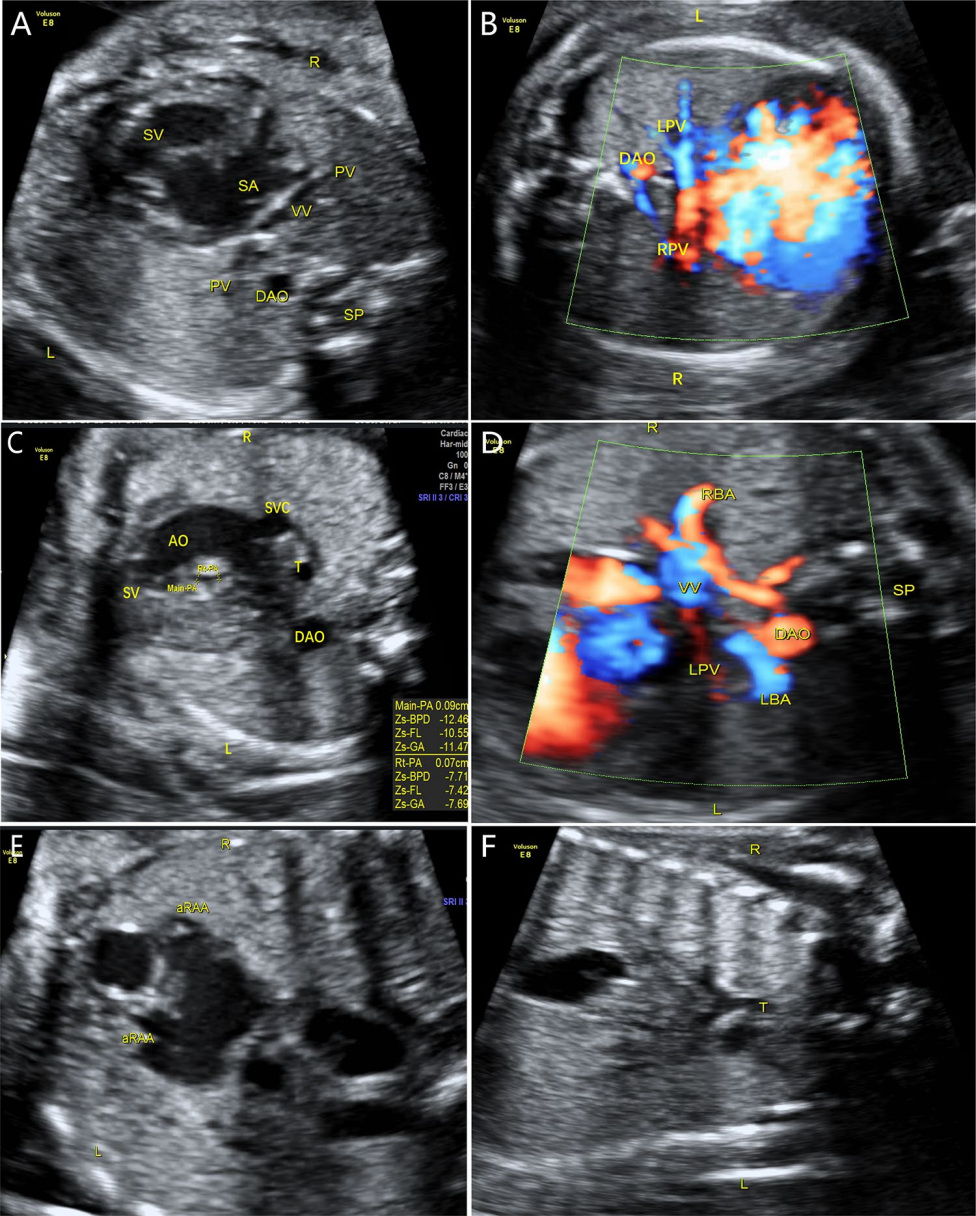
Prenatal ultrasound diagnosis of fetal infracardiac TAPVC with complex malformations. (A, B) Two-dimensional and color Doppler flow imaging showing a single atrium, single atrioventricular valve, single ventricle, and left and right PVs forming a common PV trunk, accompanied by increased posterior left atrium index. The pulmonary artery and aorta both originated from a single ventricle and pulmonary atresia was observed. (C, D) A collateral blood supply from the descending aorta in both lungs was seen in the cross section of the heart. (E) Both left and right atrial appendages were anatomically right atrial appendages. (F) Left and right main bronchi were symmetrical.
